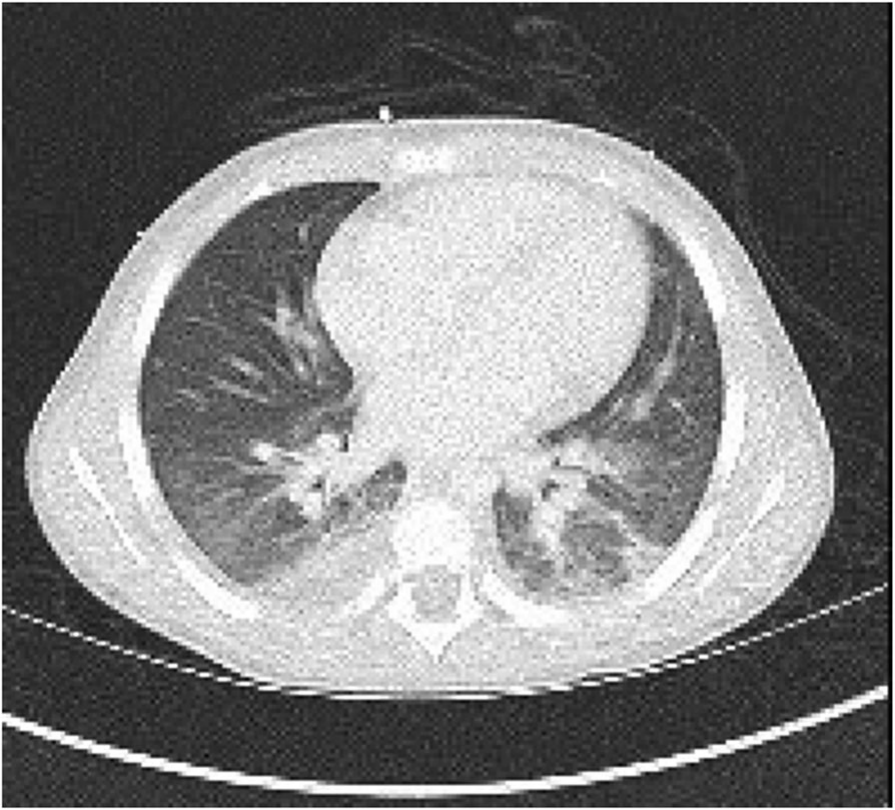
Lung bases captured by abdominal computed tomography on day 2. Shown in the lungs are bilateral lower lobe consolidation and interdigitating ground glass opacities
